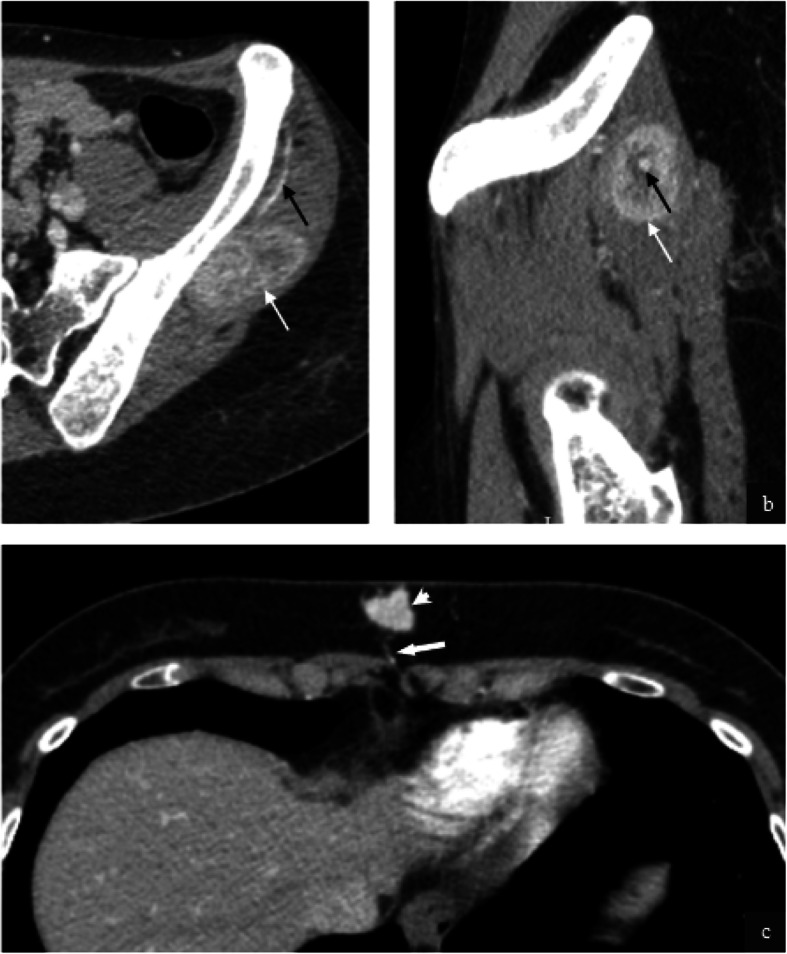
Intramuscular and subcutaneous metastases from ASPS. Post contrast axial (a) and sagittal CT (b) in a 21 year old female with an intramuscular metastasis (a & b, white arrows) demonstrates a large feeding vessel (a & b, black arrows). Post contrast axial CT in this 28 year old female patient demonstrates markedly hyperenhancing subcutaneous metastasis (c white arrowhead) inferior to the xiphisternum. Again a small feeding vessel is appreciated (c, thick white arrow)
